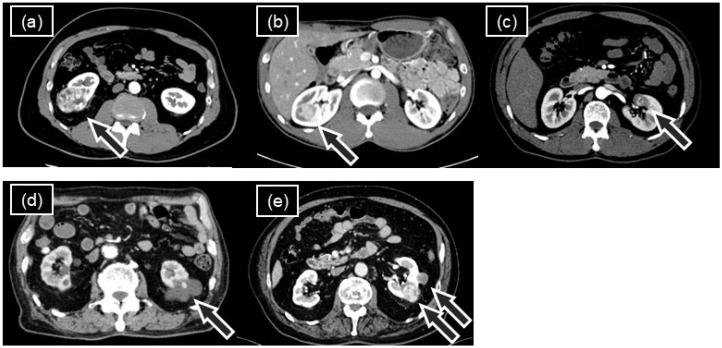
Representative axial views from preoperative contrast-enhanced abdominal computed tomography scans. In each image, arrows indicate renal tumors with complex features: (a) cT1b tumor, (b) completely endophytic tumor, (c) hilar tumor, (d) cystic tumor, (e) ipsilateral multiple tumors.
